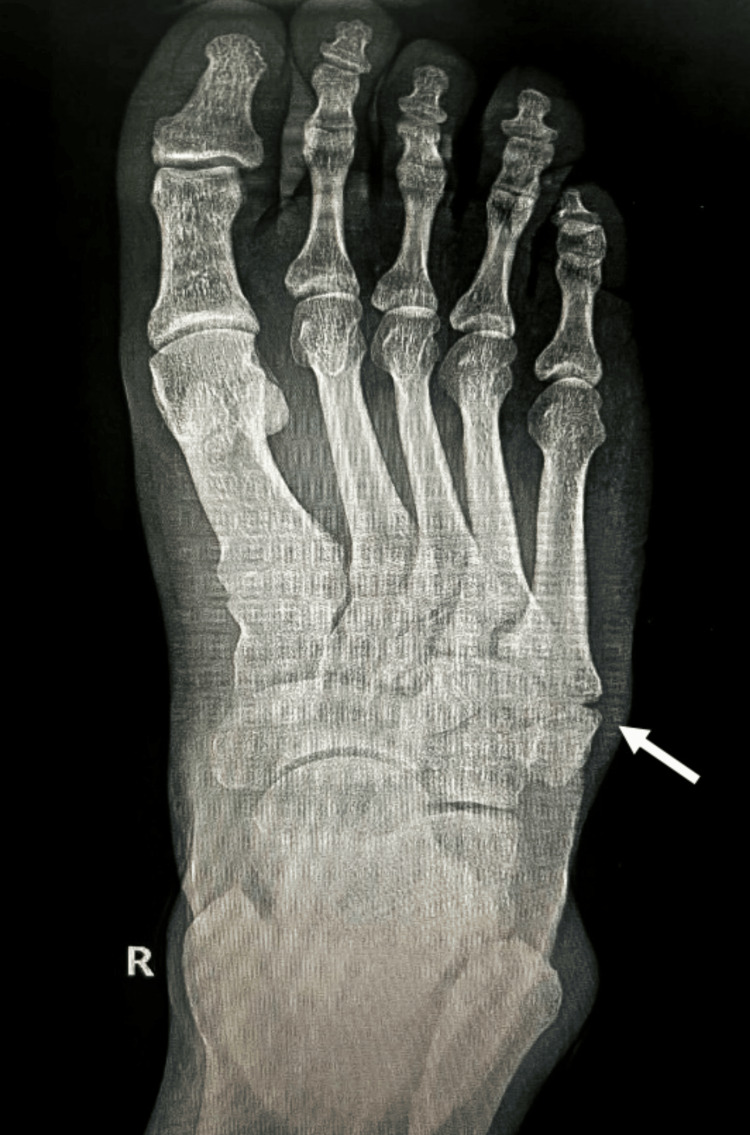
Initial X-ray of the right ankle following a twisting injury, demonstrating a fracture at the base of the fifth metatarsal
